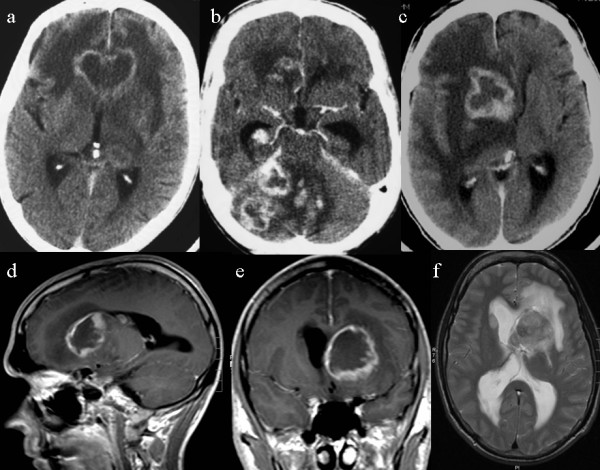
AIDS-related PCNSL in Norway: imaging findings at CT and MRI. Contrast enhanced CT in 3 patients demonstrating lesions with ring-enhancement in the frontal lobes (a), cerebellum (b) and basal ganglia (c). MRI (d-f) in another patient with lesion in the basal ganglia at T1 (d-e) and T2 (f) weighted images.
